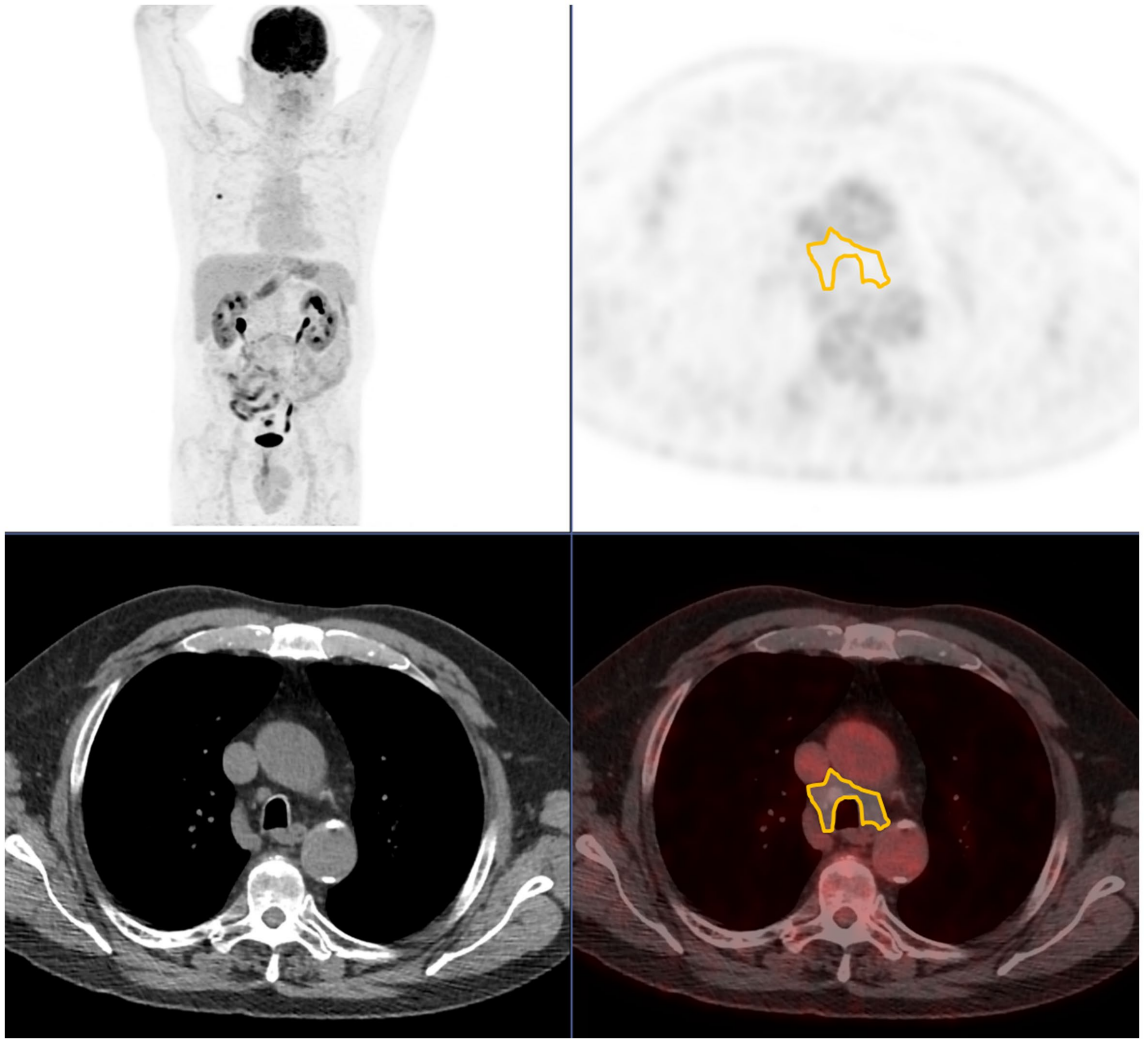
Region of interest used when no pathological lymph node uptake is seen, encompassing 4R and 4L station. From top to bottom and from left to right: maximum intensity projection; axial PET; axial CT; axial fused PET/CT.
